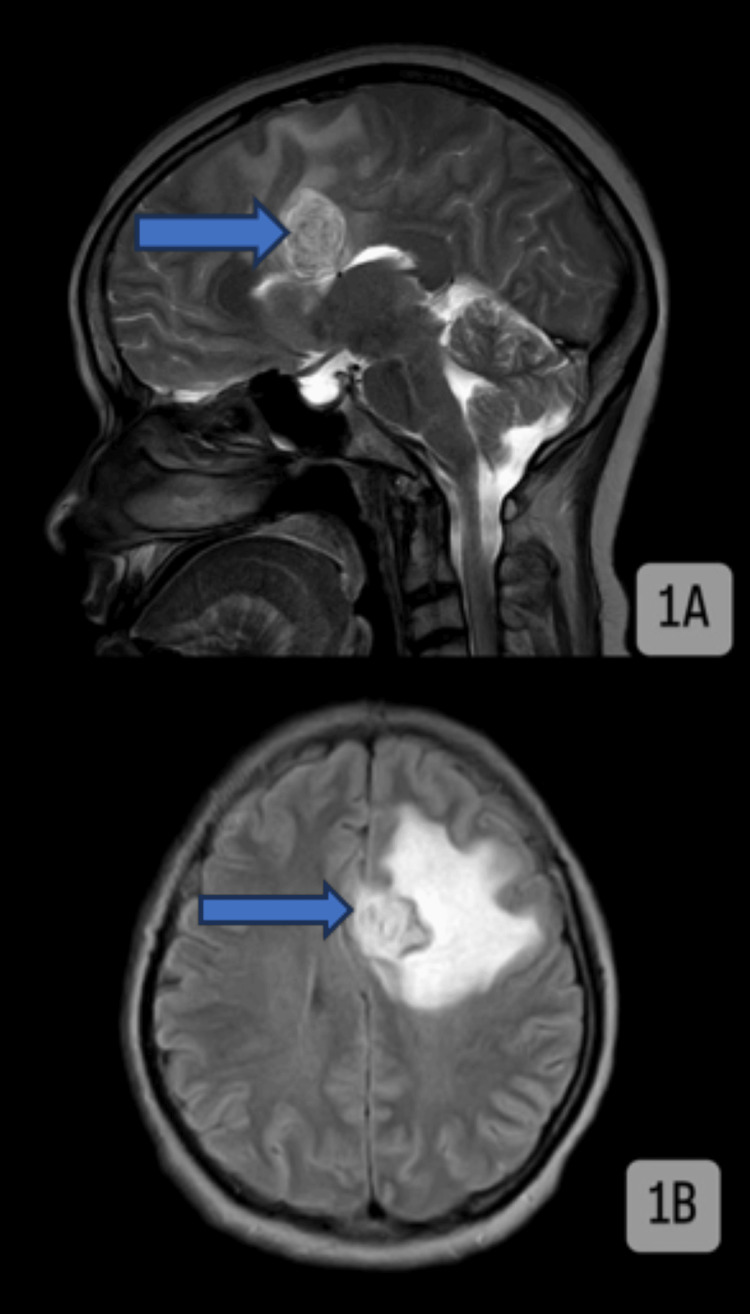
MRI of the brain (1A) Heterogeneously intensely enhancing altered signal intensity of CG appearing heterogeneously hyperintensity on T2WI/FLAIR (blue arrow; tumors typically appear hyperintense on T2WI and FLAIR image due to their different water content compared to surrounding brain tissue), hypointense on T1WI (blue arrow), showing minimal diffusion restriction on DWI and foci of blooming on SWI, suggesting hemorrhage adjacent perilesional edema is noted. The hypointensity on T1WI is suggestive of high-grade glioma (tumors in the CG often appear as hypointense areas on T1WIs). (1B) The lesion is causing a mass effect in the form of effacement of adjacent sulcogyral spaces, the frontal horn, and the body of the lateral ventricle with displacement of the falx toward the right, compressing the body and the isthmus of the CC. CC, corpus callosum; CG, cingulate gyrus; DWI, diffusion-weighted imaging; FLAIR, fluid-attenuated inversion recovery; SWI, susceptibility-weighted imaging; T1WI, T1-weighted image; T2WI, T2-weighted image
